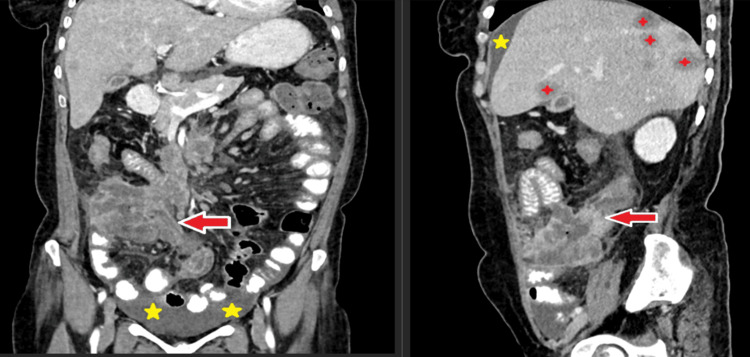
Malignant thickening of bowel wall with secondary hepatic and lymph nodal metastasis and ascites (case 2). Contrast-enhanced computed tomography (CECT) abdomen coronal and sagittal sections show marked heterogeneous enhancing thickening of the distal ileum, ileocecal junction, and part of the cecum, with loss of bowel wall layer definition (red arrows) involving a segmental length, along with secondary ascites (five-point yellow stars) and hepatic metastasis (four-point red stars).
